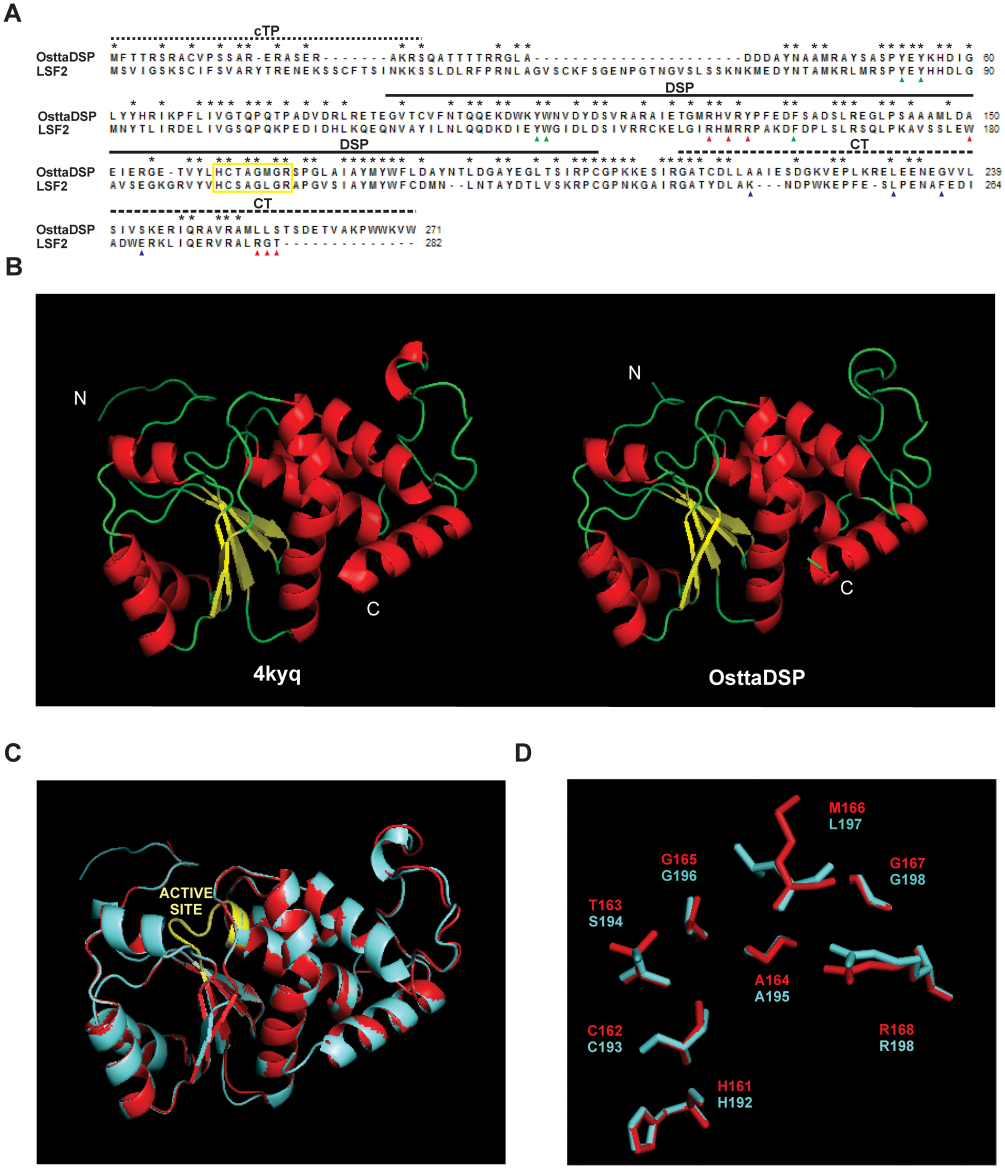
Amino acidic sequence alignment and homology modelling of OsttaDSP. A) Amino Acidic Sequence Alignment of OsttaDSP with *A*. *thaliana* LSF2. The yellow box highlight the active site. cTP, chloroplast transit peptide; DSP, dual specific phosphatase and CT, C-terminal. Residues involved in glucan binding in *A*. *thaliana* LSF2 are indicated within the aromatic channel (green arrowheads), SBS2 (red arrowheads) and SBS3 (blue arrowheads). Residues in the aromatic channel are conserved between OsttaDSP and LSF2, however, residues in SBS2 and SBS3 are not. B) Structural model of *A*. *thaliana* LSF2 (4kyq, left) and the proposed model for OsttaDSP (right). α- helix are indicated in red, β- sheets in yellow and loops in green. N, N-terminal and C, C-terminal. C) Superposition of LSF2 (cyan) and OsttaDSP (red) structures. The active site is shown in yellow. D) Superposition between OsttaDSP model and LSF2 structure showing the residues involved in the active site. OsttaDSP residues are shown in red and LSF2 residues are shown in cyan.
